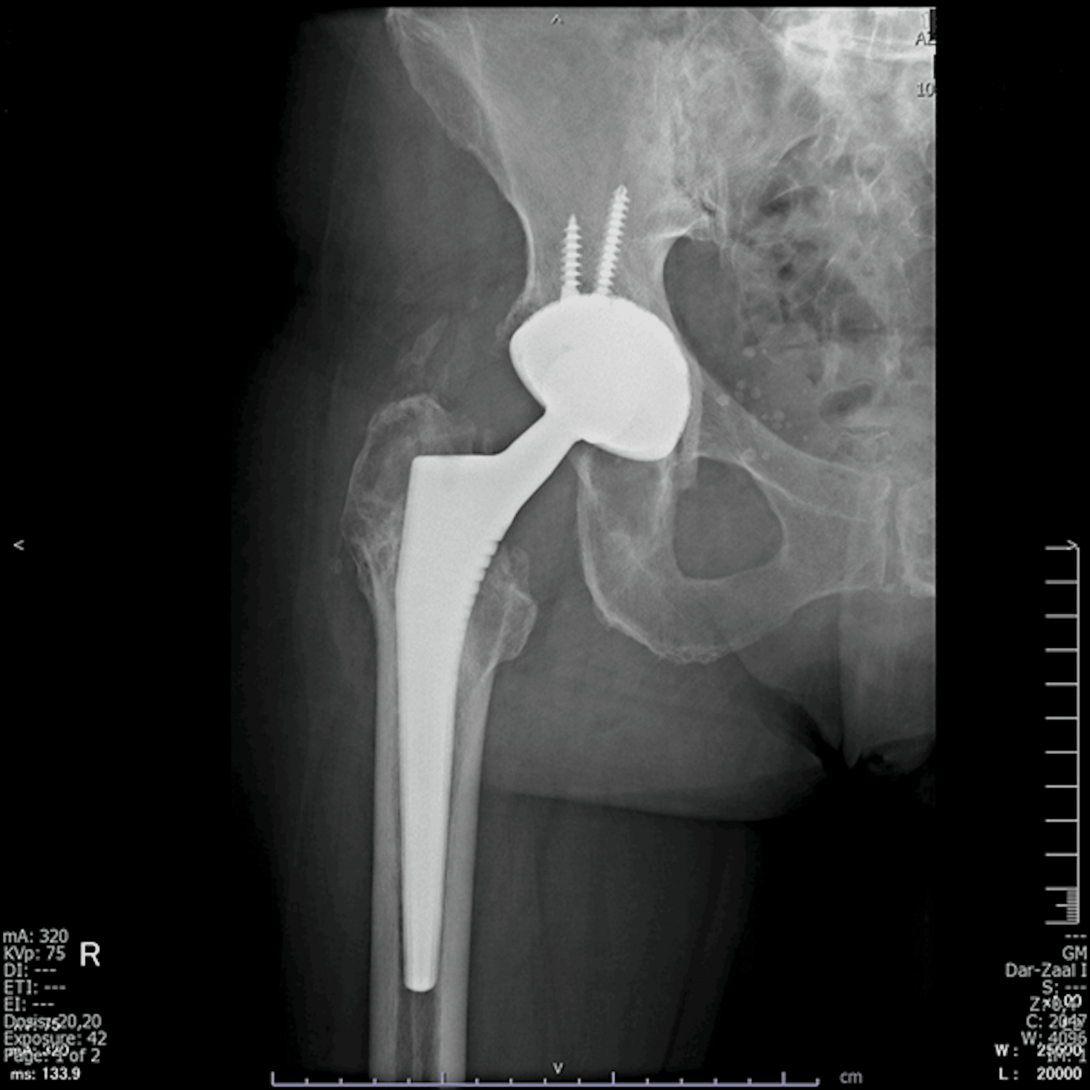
Anteroposterior right hip X-ray of the same patient one year after revision surgery.
